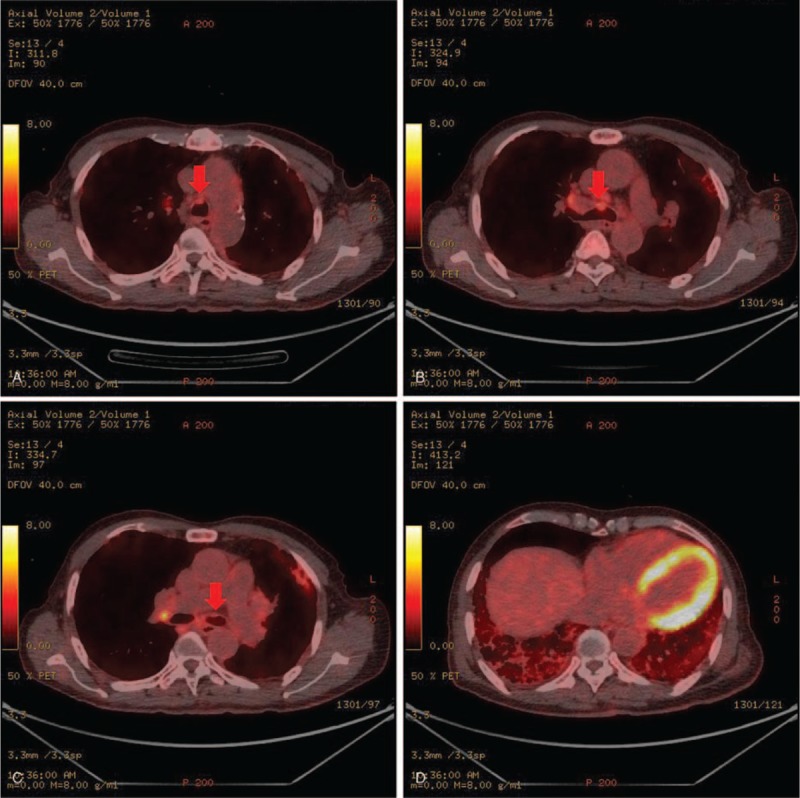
(A–C) Positron emission tomography CT showed FDG avid soft tissue density nodules at trachea and bronchus wall. (D) Diffuse FDG uptake was noted at both lower lung fields. CT = computed tomography, FDG = fluorodeoxyglucose.
